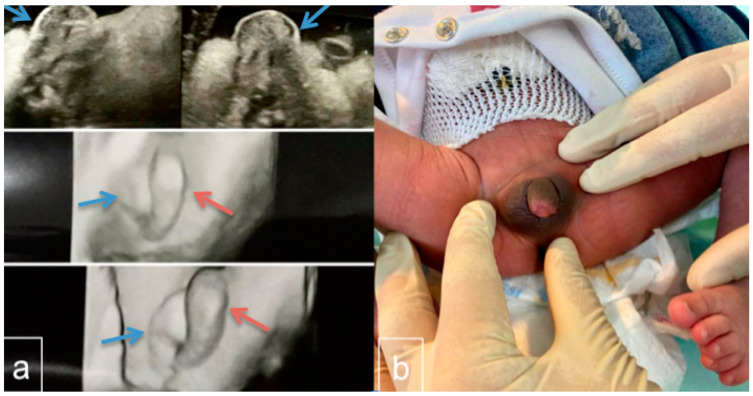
(a) Ultrasound scan, 2D and 3D, of the fetal genitalia done in the second trimester. Visualization of the vulva, clitoris, and labia is considered to indicate a female fetus whereas demonstration of the scrotum, penis, descended testicles, and penile midline raphe is interpreted as indicative of a male. In the ultrasound image, there is a labial/scrotum-like formation (blue arrow) and a median prominence similar to the phallus/clitoris (red arrow). (b) Infant 24 h after birth with ambiguous genitalia. The phallus was 1.4 cm in length with a blind dimple on the glans and a urethra that opened ventrally at the base, consistent with Prader 4 hypospadias. The clitoral index was 0.7. The anogenital ratio was 0.78. No gonads were palpable in the labial/scrotal tissue. Pelvic imaging did not identify a uterus or ovary, but only small formations in the inguinal duct.
